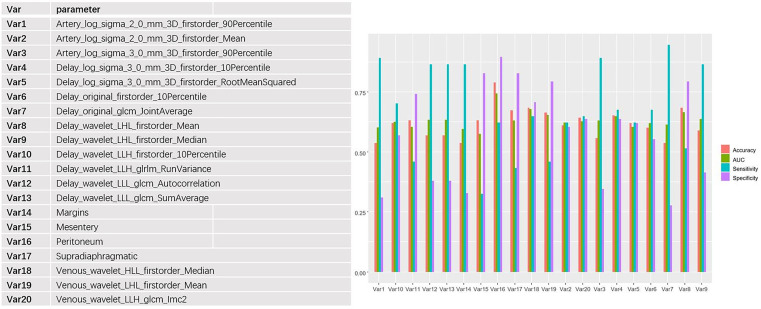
Independent predictive efficacy of the 20 features with the best performance (including CT imaging features and texture features), among which the peritoneal transition form had the best diagnostic efficacy, with AUC, accuracy, specificity, and sensitivity of 0.74, 0.79, 0.90, and 0.62, respectively.
